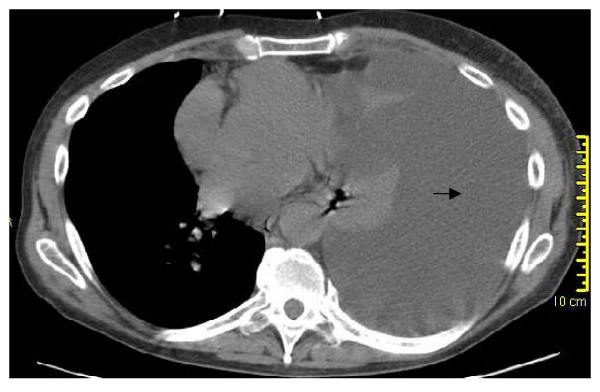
CT chest: massive pleural effusion on left side (arrow).
